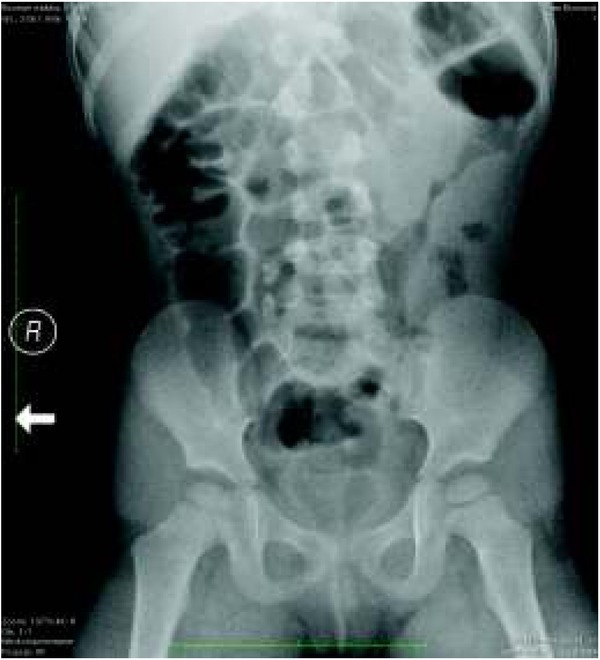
Abdominal X-ray. Two stones in the right ureter and suspected right renal pelvic stone. Ryc. 2. Zdjęcie przeglądowe jamy brzusznej. Widoczne dwa złogi w moczowodzie prawym i podejrzenie złogu w miedniczce nerki prawej.
